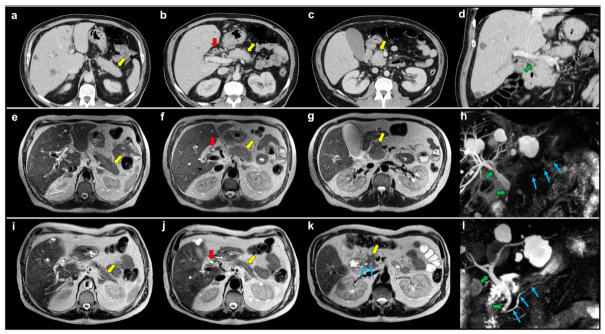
IgG4-SC and diffuse AIP in a male patient presenting with jaundice, elevated bilirubin and right upper quadrant pain. (a–d) Axial and coronal CT images in the portal phase show signs of diffuse AIP with pancreatic enlargement, loss of lobulations and a subtle hypodense “halo” (yellow arrows). Associated IgG4-SC is evidenced by smooth circumferential wall thickening of the common hepatic duct (CHD) (b, red arrow), associated with distal CBD stricture and upstream biliary dilatation (d, green arrow). (e–h) Subsequent MRI was performed. (e–g) Axial T2WI shows mild hyperintensity of the pancreatic parenchyma with a peripancreatic hypointense “halo” (yellow arrows) and confirms the bile duct wall thickening (f, red arrow). Note also the severe strictures of the MPD and intrapancreatic CBD, resulting in poor visualisation of both ducts. (h) Coronal 3D MRCP with MIP reconstruction demonstrates hilar stenosis at the biliary confluence and distal CBD stricture (green arrows) with upstream dilatation, consistent with type 3 IgG4-SC. The MPD cannot be clearly identified at the pancreatic head due to severe stenosis, and shows skipped strictures in the pancreatic body and tail, without upstream dilatation (blue arrows). (i–l) Follow-up MRI after two months of steroid therapy. (i–k) Axial T2WI shows a decrease in pancreatic volume with resolution of the inflammatory changes (yellow arrows) and improvement of the CHD wall thickening (j, red arrow). (l) Coronal 3D MRCP with MIP reconstruction demonstrates resolution of both the hilar stenosis at the biliary confluence and the distal CBD stricture (green arrows), accompanied by normalisation of the MPD calibre, which is now clearly visible along its entire course (blue arrows).
